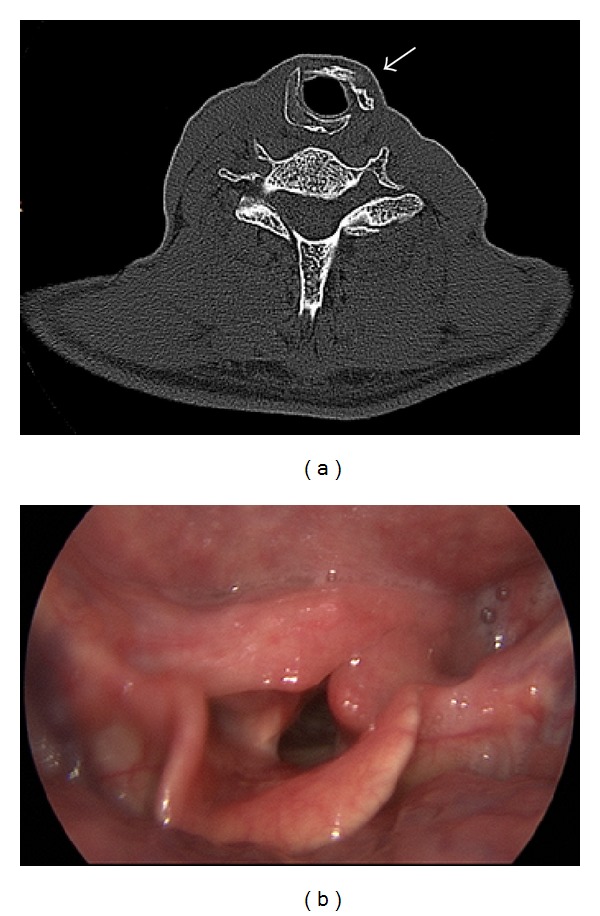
(a) CT scan showing thyroid cartilage used for reconstruction (arrow). (b) Videolaryngoscopic image showing adequate airway passage.
